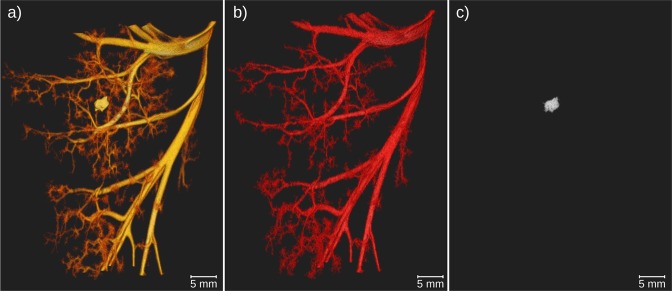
3D visualization of segmented CT data to visualize solely the hyperdense structures. (a) The blood vessels and kidney stone were segmented from the unfiltered CT scan. The differentiation of the two materials is not possible; (b) blood vessels were segmented from the iodine KES data, in which the kidney stone is not visible; (c) by performing inverse KES the calcium within the kidney stone becomes visible again whilst the iodine-filled blood vessels are subtracted from the image.
